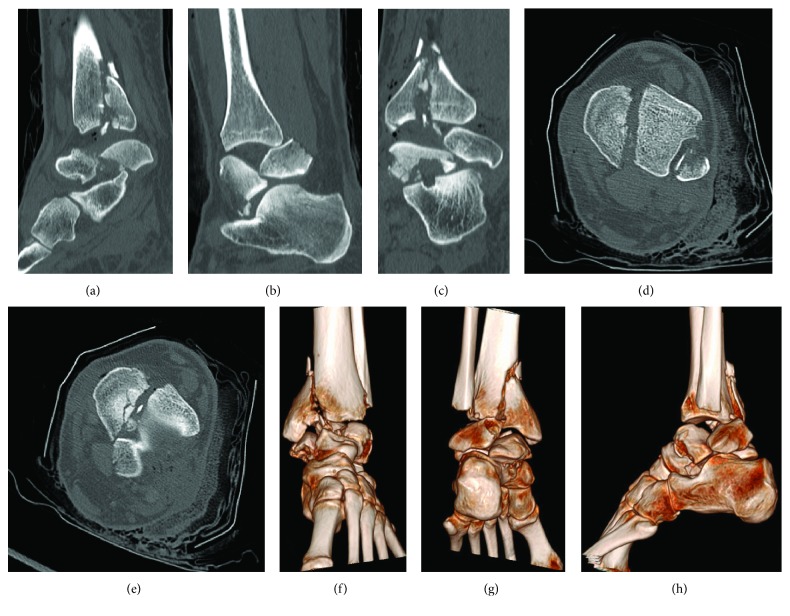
Preoperative CT scan of the left ankle with (a) the sagittal view of the pilon fracture and the split fracture of the talus, (b) distal fibula pushing the talus fragment anterior, (c) frontal view of the pilon fracture and the talus split fracture, (d) axial view with the fibula in the incisura, (e) fibula splitting the tibial plafond, and reconstruction of the left foot in (f) frontal anterior, (g) frontal posterior, and (h) sagittal lateral views.
